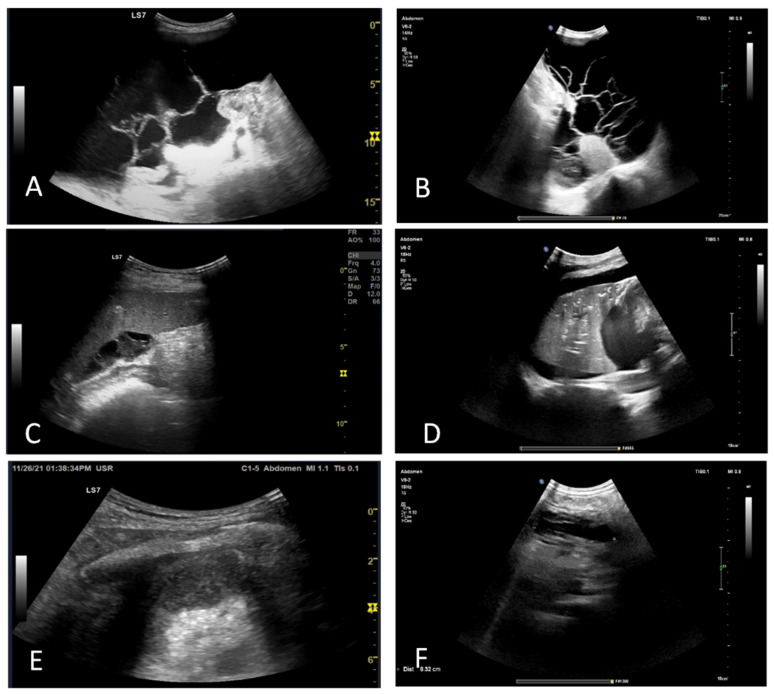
Abdominal and thoracic ultrasound in evolution. (A,B): Peritoneal effusion with septa. (C):Perisplenic cloasonated peritoneal effusion. (D): Pleural effusion of the inferior lung lobe, with air bronchograms. (E,F): Left subphrenic effusion, showing deep enhancement, suggestive of infection (abscess).
